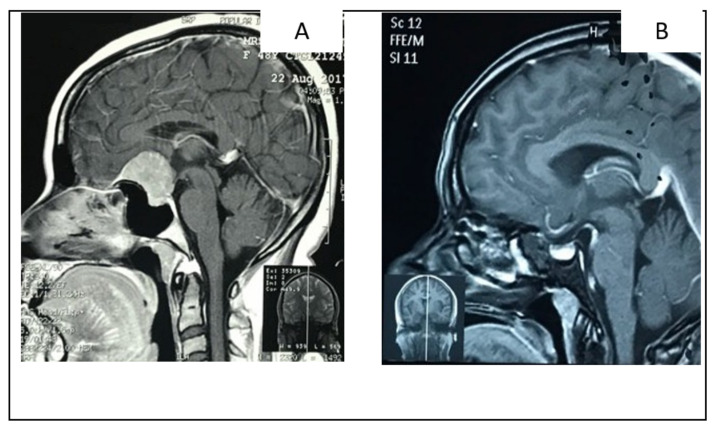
(A) Sagittal MRI with contrast in sagittal view showing small- to medium-size TSM, compressing of the pituitary gland. (B) Sagittal MRI with contrast in sagittal view showing no residual tumor following extended endonasal approach.
